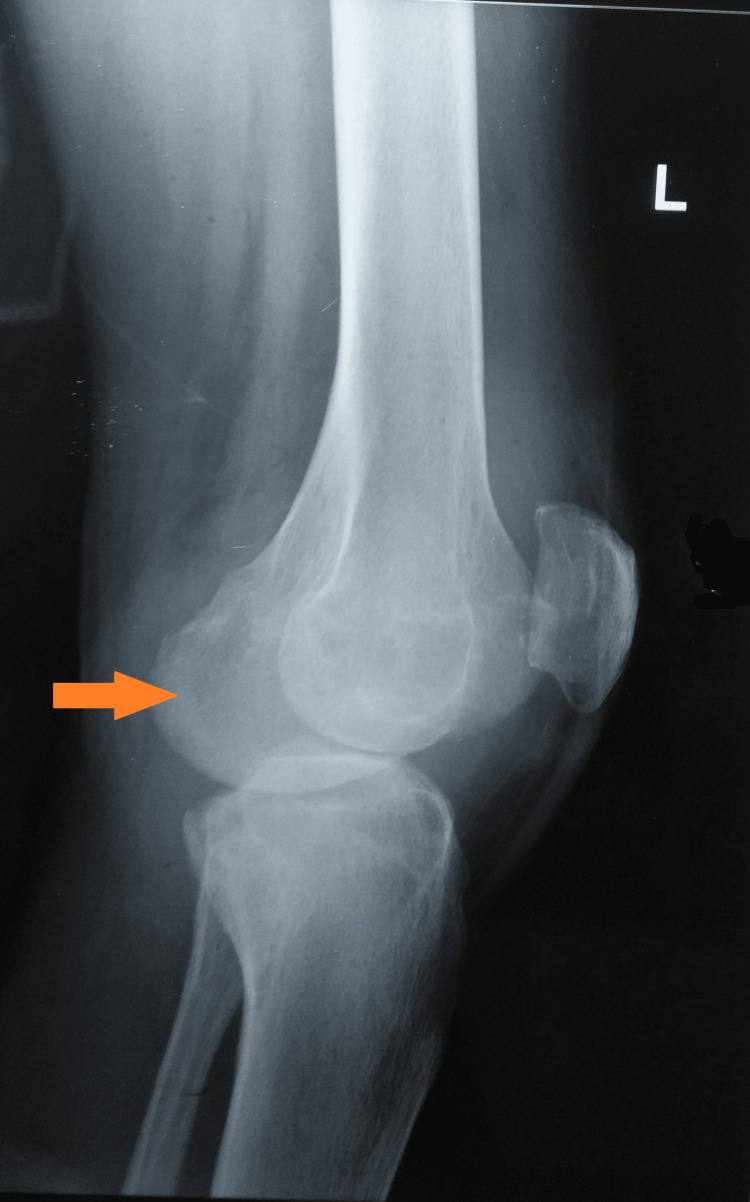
Plain radiograph (lateral view) of the left knee at the nine-month follow-up
